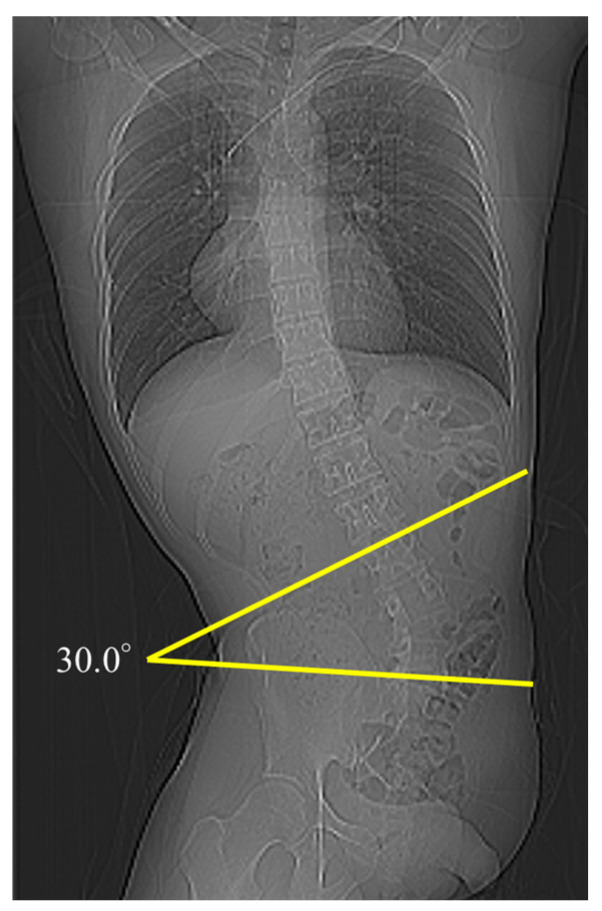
CT scout image of a patient who had undergone hemipelvectomy.
